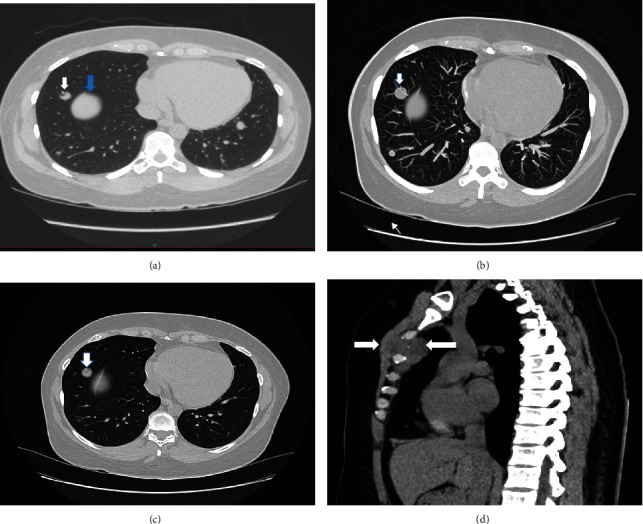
Initial CT scan at the time of motor vehicle accident in November 2019 (a) shows multiple pulmonary nodules the largest measuring 1.2 cm (white arrow) in the anterior right lower lobe (RLL). The blue arrow in the CT scan shows the top of the liver. In March 2020, CT scan of the chest (b) showed the dominant RLL lesion now measuring 2.0 cm depicted with the thicker arrow and a new smaller posterior RLL nodule depicted with the thinner arrow. After starting tamoxifen, the CT scan of the chest in November 2020 (c) shows stability of the dominant anterior RLL measuring 1.8 cm and the posterior RLL nodule is improving. (d) Is the sagittal image of the chest CT in March 2020 revealing the sternum mass that measured 5.7 × 3 cm with invasion into the cortex of the bone causing destruction of the bone.
